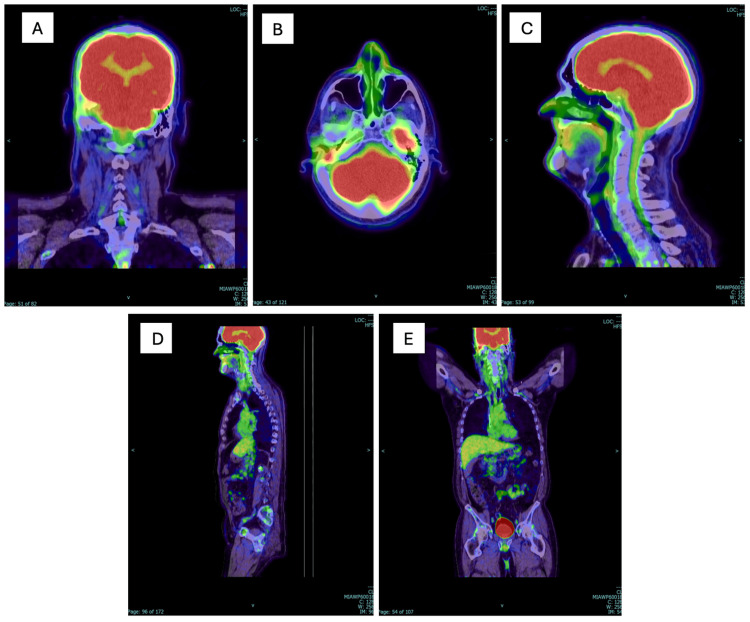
Follow-up PET/CT from skull base to mid-thigh performed in 2024. (A) Fused coronal images of the head and neck. (B) Fused axial images of the head and neck. (C) Fused sagittal image of the head and neck. (D) & (E) Sagittal and coronal fused images. PET/CT: Positron emission tomography/computed tomography
